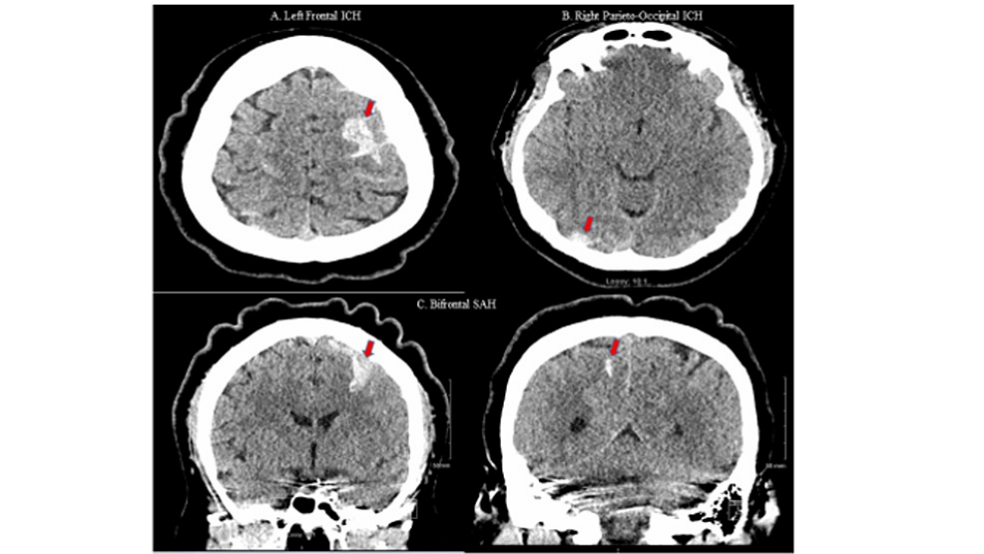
CT Head demonstrating (A) left frontal intraparenchymal, (B) right parieto-occipital intraparenchymal, and (C) multifocal bilateral subarachnoid hemorrhages (arrows)
